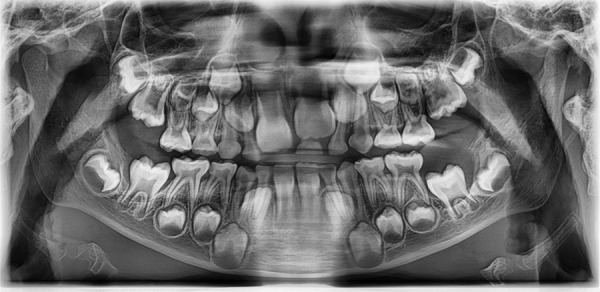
Panoramic radiography revealing an expansile radiolucent lesion related to the first molar in the left side.
